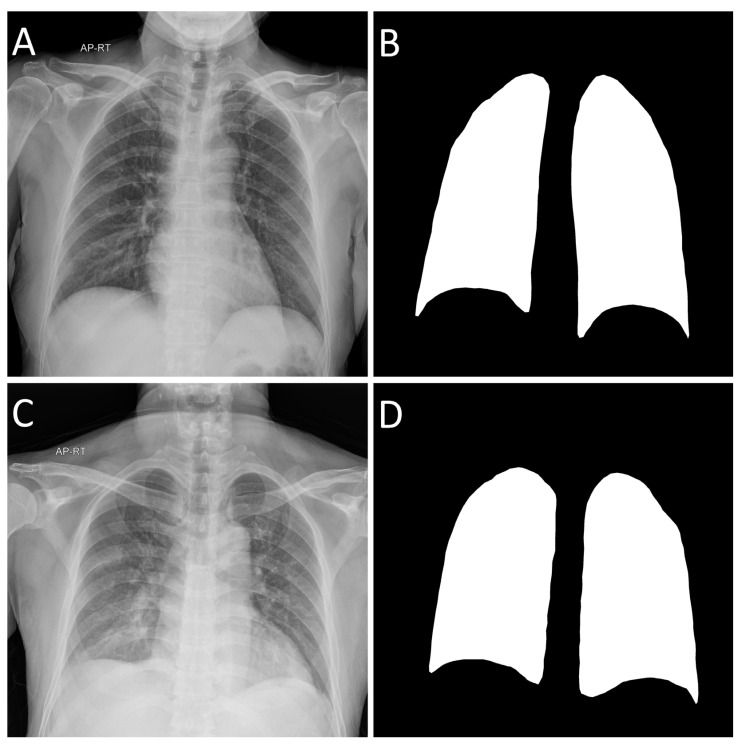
Chest X-rays and ROIs for lungs collected for COVID-19 and pneumonia. (A) COVID-19 image, (B) ROI mask image from COVID-19 image, (C) pneumonia image, and (D) ROI mask image from pneumonia image. ROI, region of interest.
